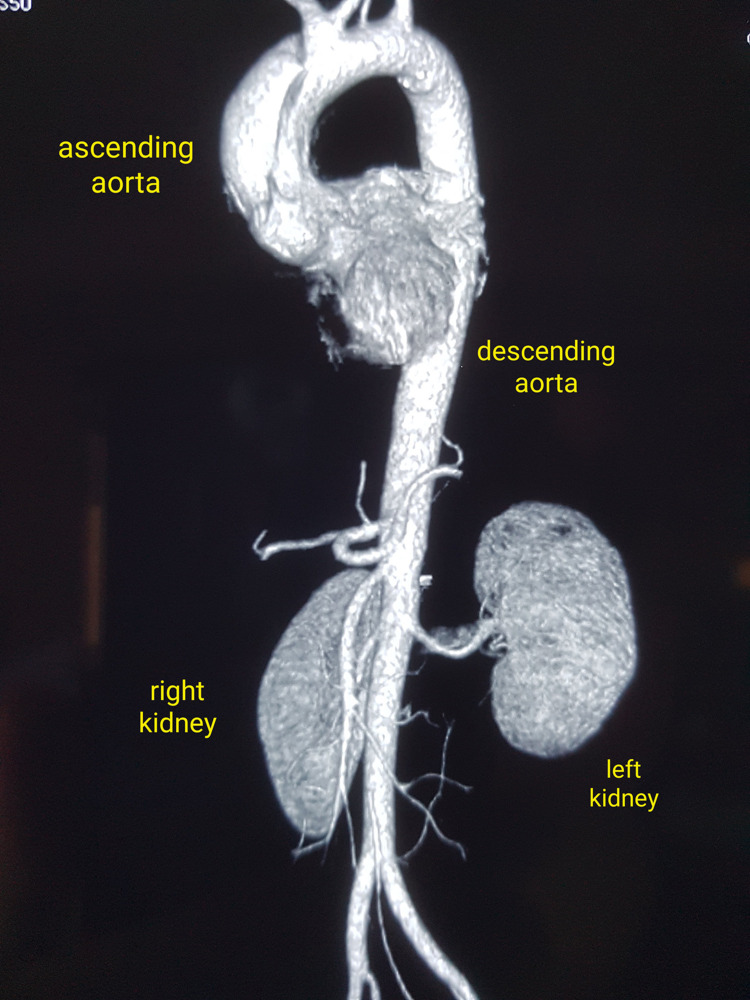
CT aortogram showing dissection in the anterior wall of the ascending aorta
